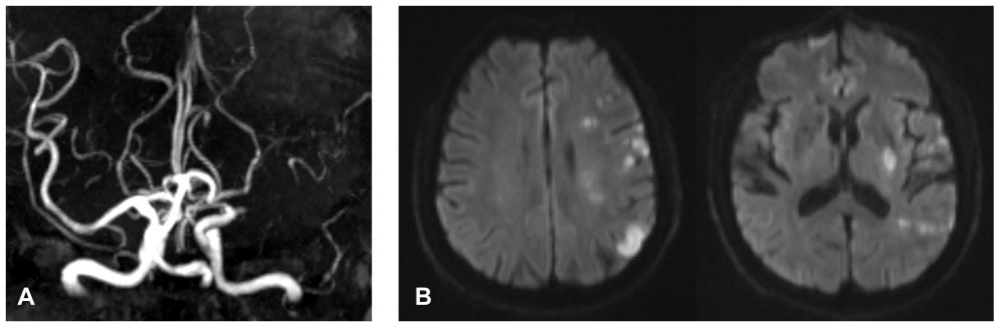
Endpoint MRA and DWI images of one of the patients with recurrent ischemic stroke, who had a SIR of 0.95 for left MCA stenosis at baseline. MRA (A) showed left MCA occlusion and DWI (B) showed multiple and small subcortical/cortical lesions within left MCA territory. MRA, magnetic resonance angiography; DWI, diffusion-weighted imaging; SIR, signal intensity ratio; MCA, middle cerebral artery.
